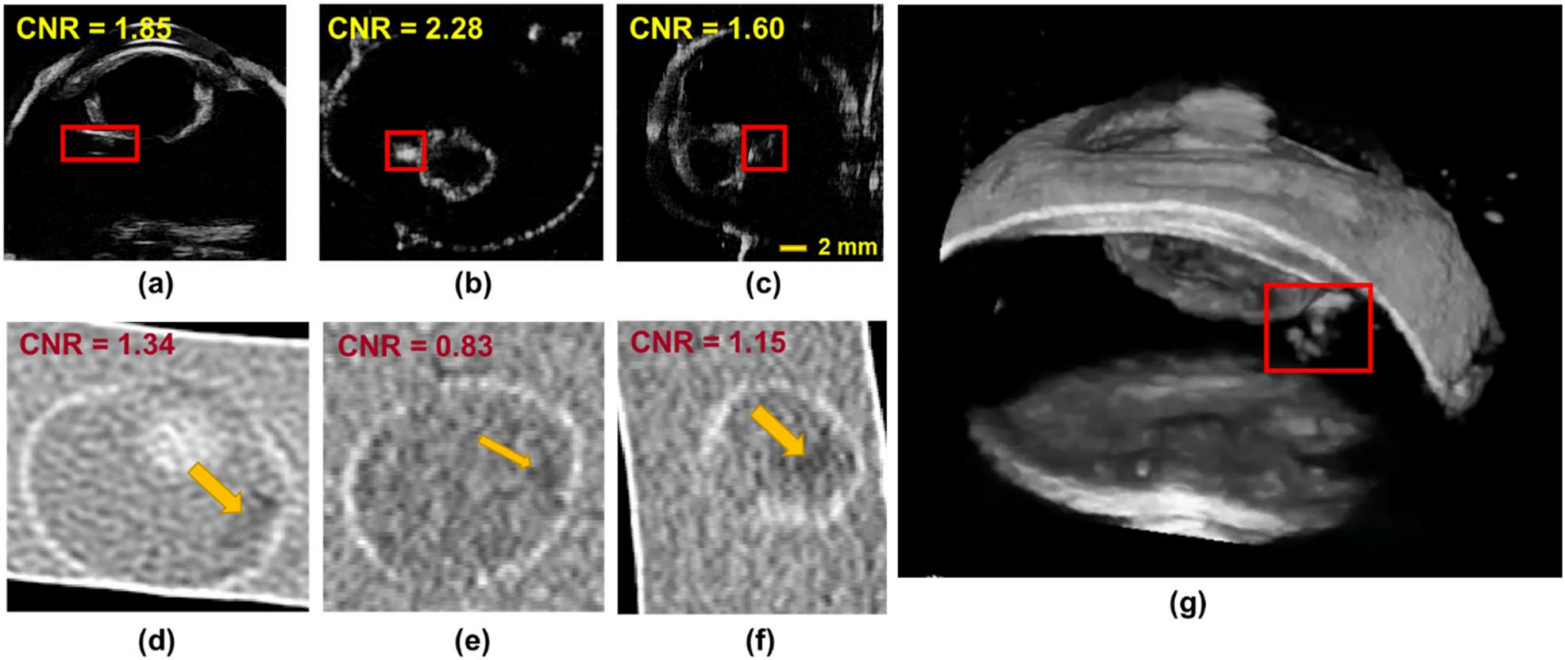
Visualization of plastic IOFB in 3D-UBS and CT. (a)–(c) and (d)–(f) show 2D views of the same plastic IOFB using 3D-UBS (red box) and CT (yellow arrow), respectively. Due to the vitreous being anechoic to ultrasound, 3D-UBS shows superior contrast compared to CT in all three planes. Out-of-plane contrast of the plastic IOFB using CT is very poor compared to 3D-UBS, making it difficult to identify the IOFB properly. En face views (b) vs (e) shows the highest (2.75×) contrast-to-noise ratio (CNR) improvement. (g) shows 3D visualization of the entire plastic IOFB. Plastic IOFB causes acoustic shadowing due to high impedance mismatch, occluding some parts of the IOFB as seen in (g).
